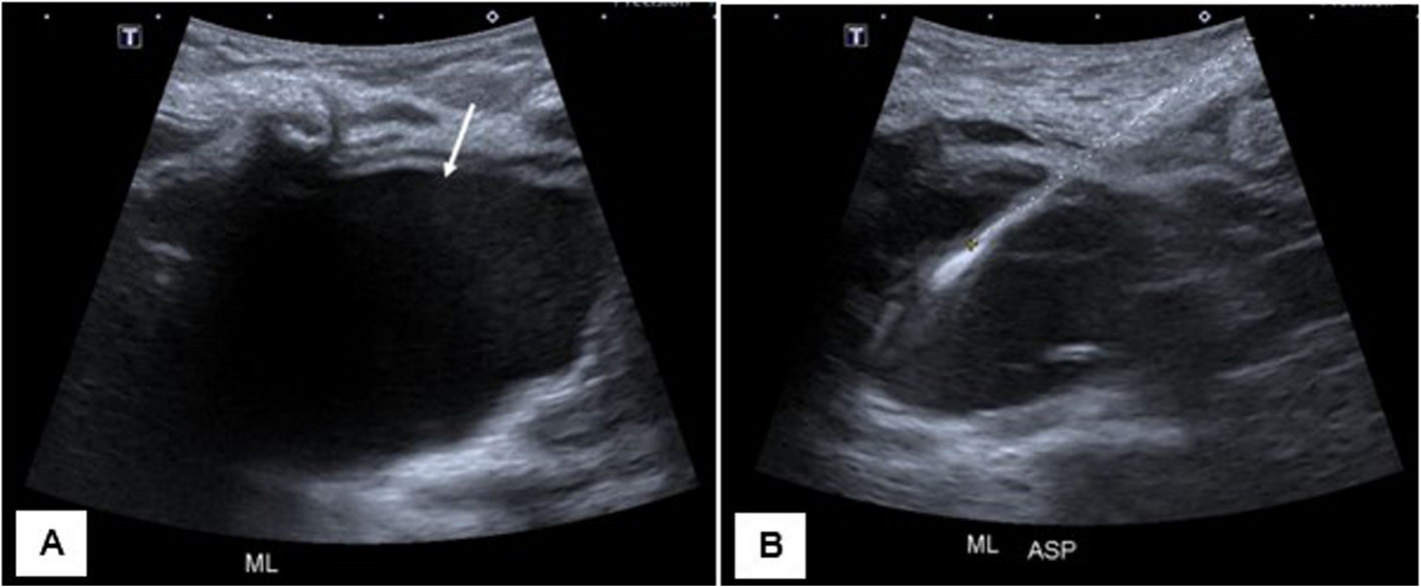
Gray-scale sonographic images of the upper mediastinum in the same patient show a large anechoic cystic structure (arrow) with no internal flow on color Doppler (not shown) (a). Approximately 12 ml of clear mucinous fluid was aspirated from the lesion (b)
